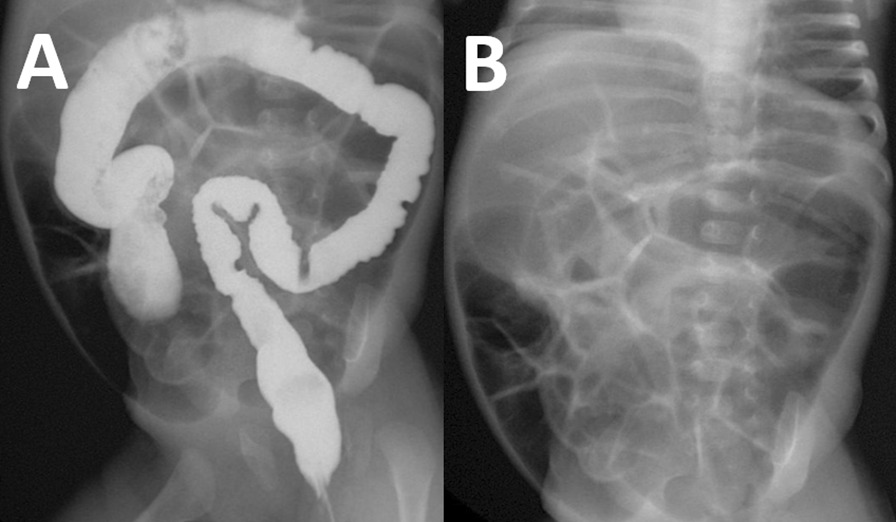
A Transition point cannot be visualized via barium enema (day 4 after hospitalization). B Anteroposterior radiograph demonstrating significant enterocolonic flatulence (day 4 after hospitalization)
